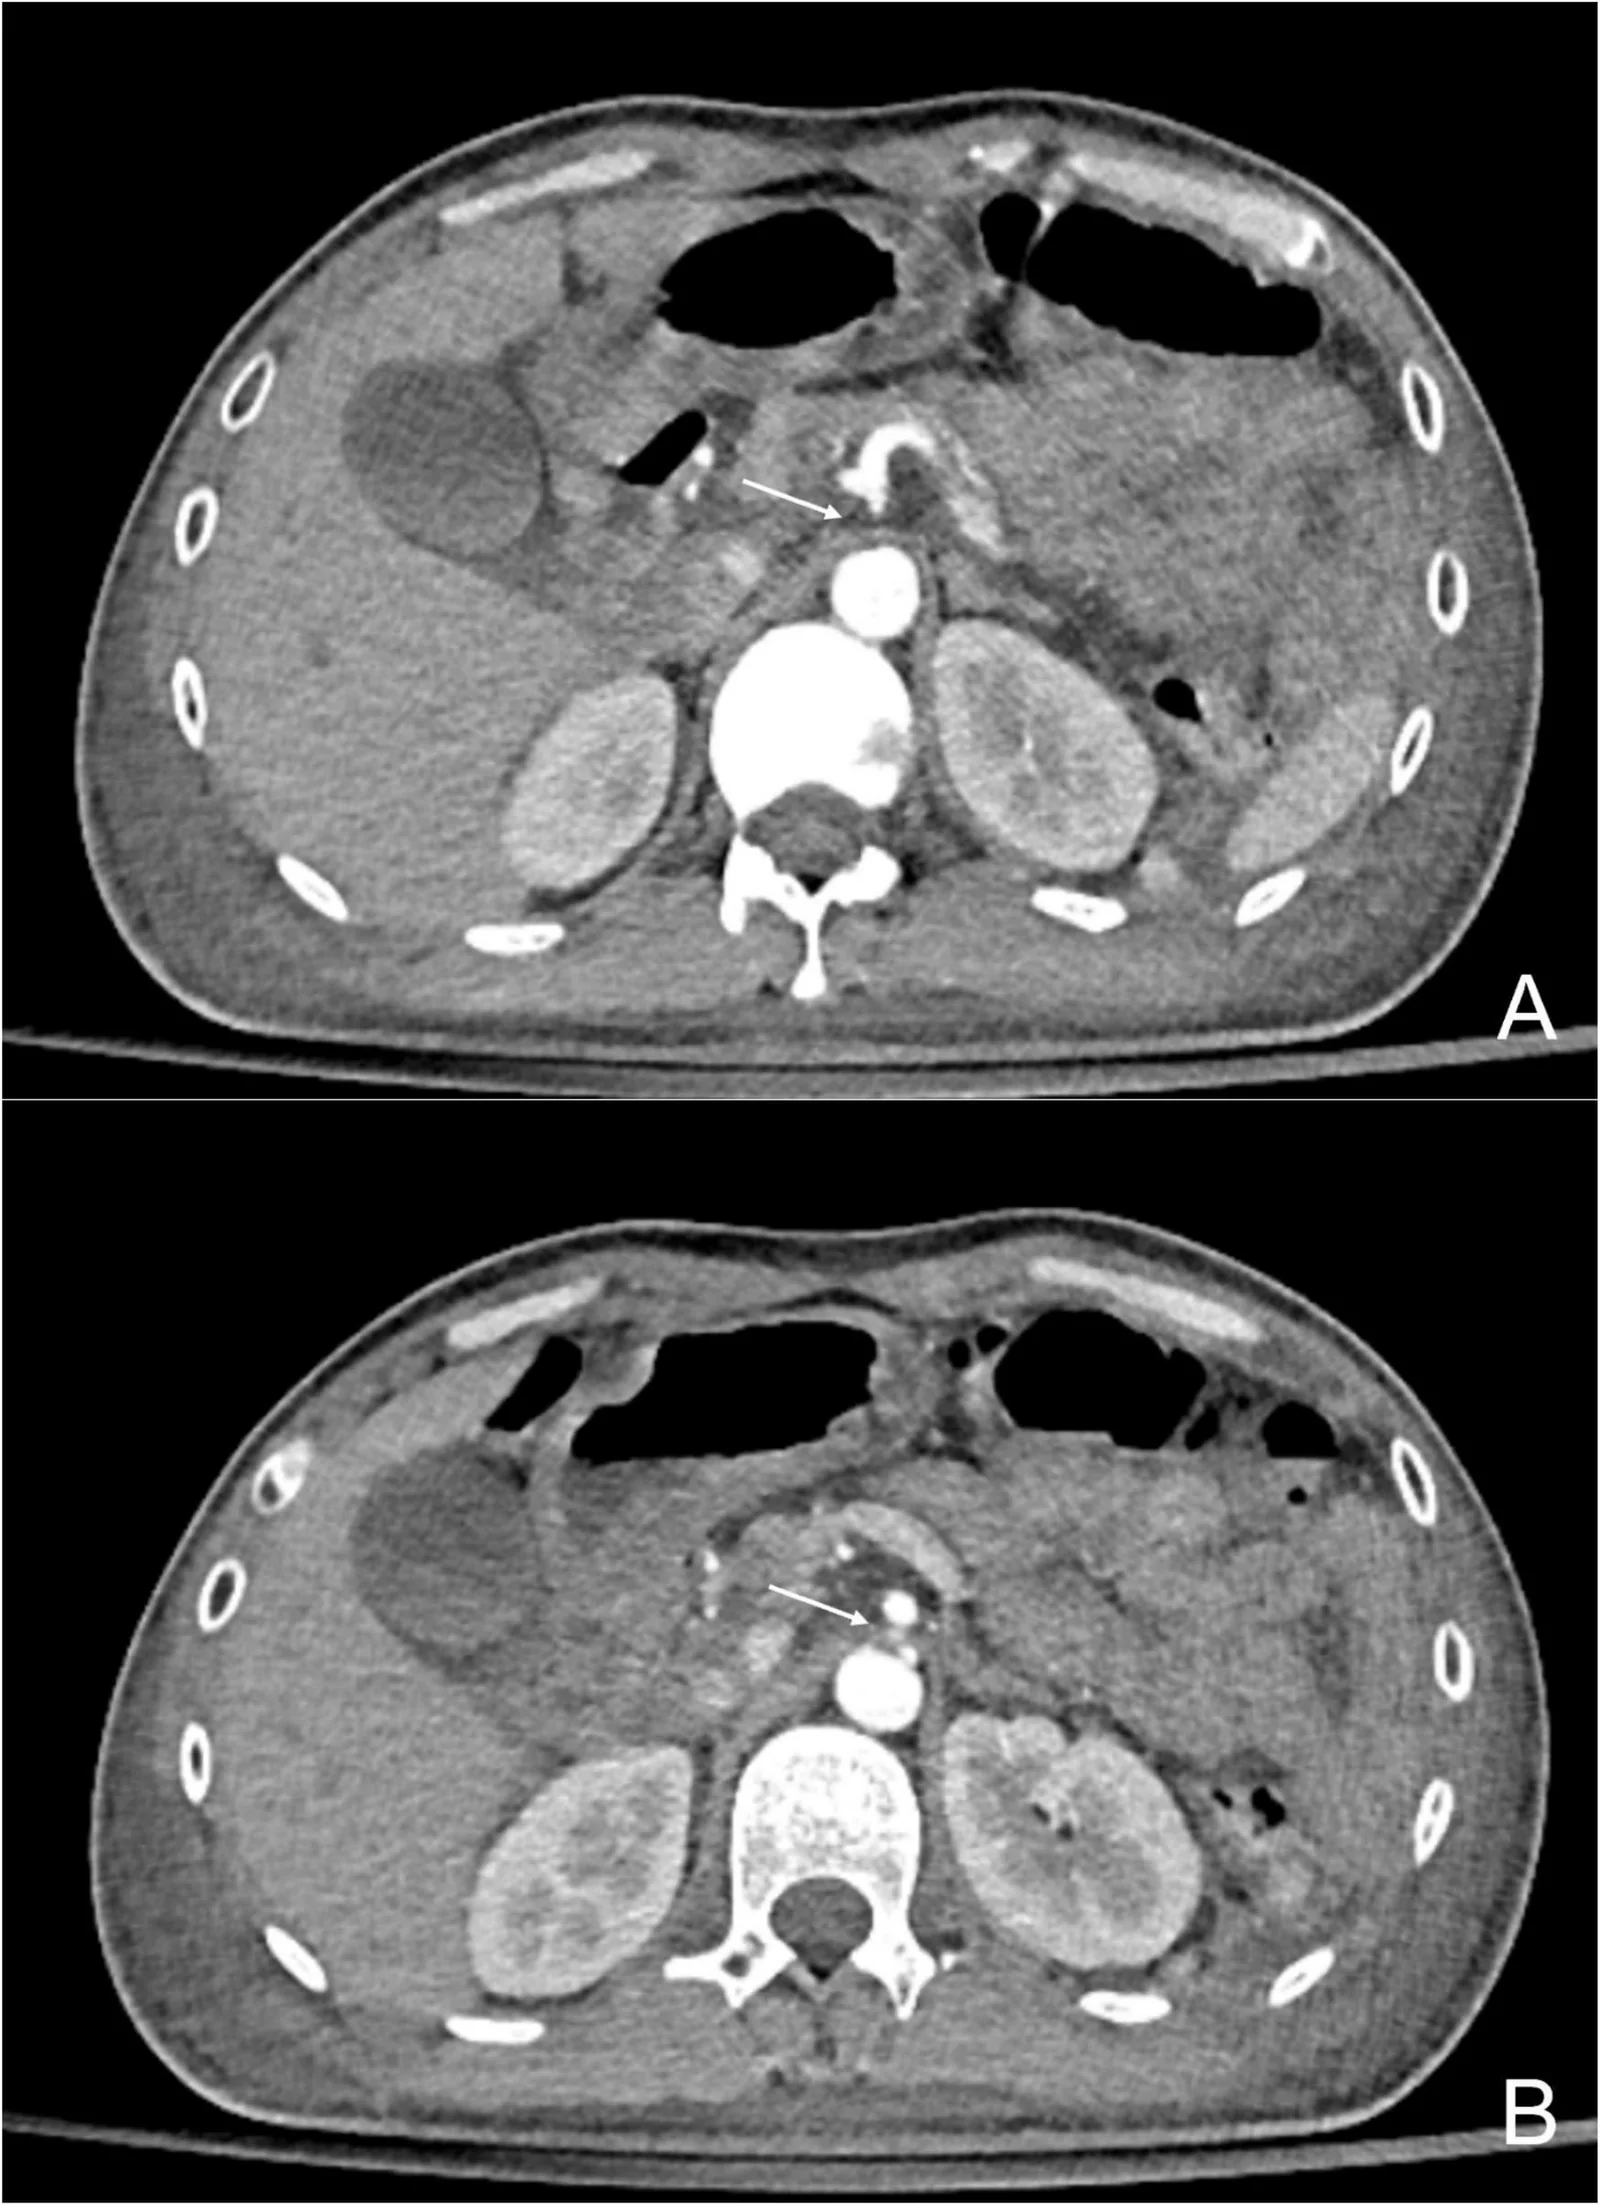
(A, B) Results of full abdominal enhanced CT scan. The proximal part of the celiac artery was narrowed and occluded. Abdominal and pelvic hemorrhage and effusion, swelling of the anterior sacral soft tissue and the bilateral lumbar muscles, and slight swelling and thickening of some small intestines and intestinal walls.
